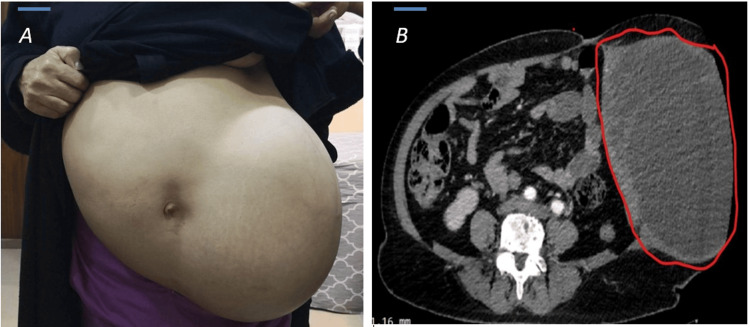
(A) Preoperative clinical photograph showing a large mass occupying the left abdominal wall. (B) Abdominal computed tomography revealed a large (30 x 37 x 20 cm) complex multiloculated cystic lesion on the left side of the abdominal wall.
